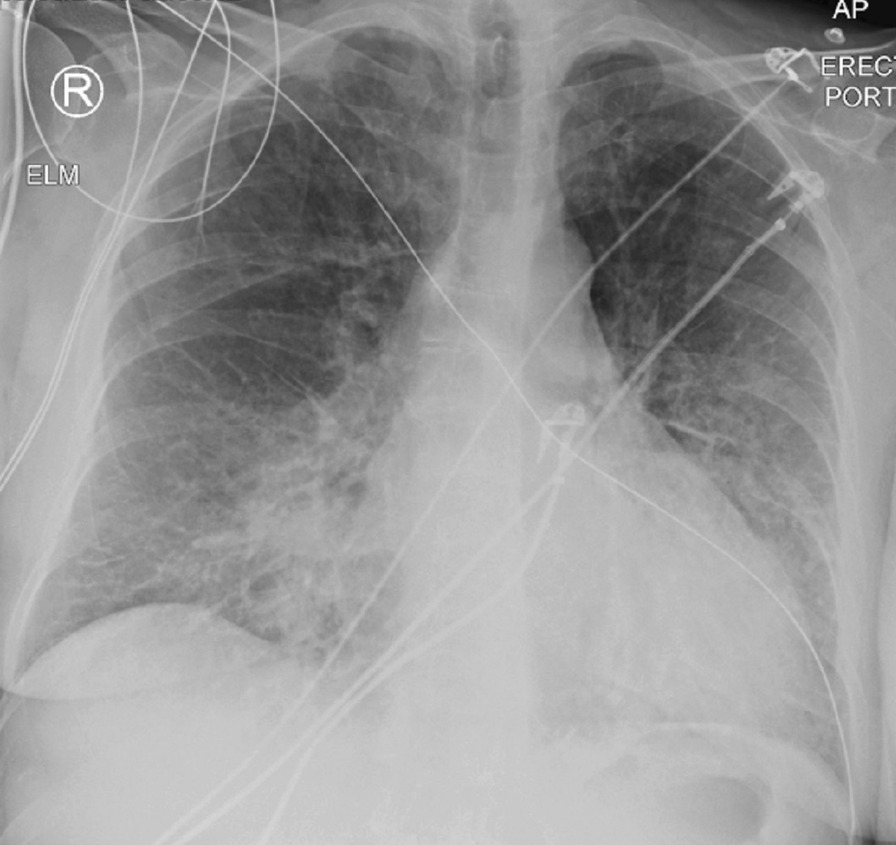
Case 9: Figure 2. Portable chest radiograph. Bilateral, peri-hilar airspace opacities and Kerley B lines consistent with acute pulmonary edema
